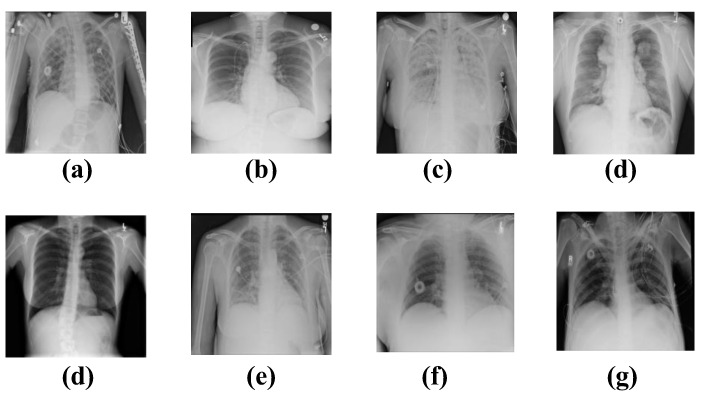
Eight common thoracic diseases observed in chest X-rays. (a) atelectasis, (b) cardiomegaly, (c) effusion, (d) infiltration, (e) mass, (f) Nodule, (g) pneumonia, and (h) pneumothorax.
